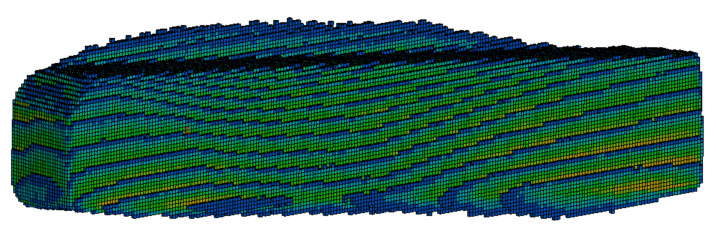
Unequal components of the K2F3 sample immediately after the CBCT scan.
